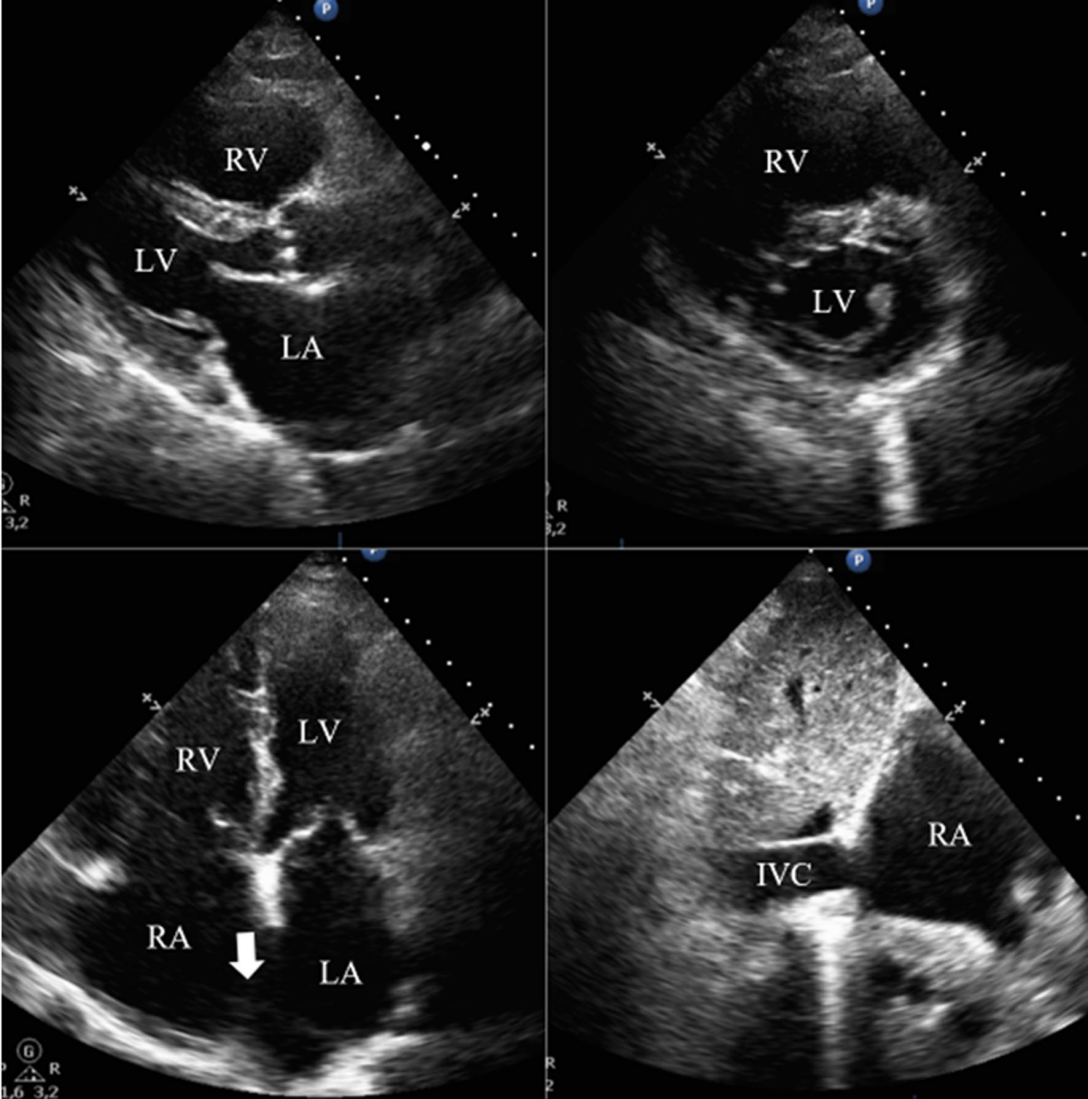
TTE imaging showing severe dilation of right cardiac chambers and RV volume overload secondary to a secundum ASD. During hospitalization, a TTE was performed demonstrating an ostium secundum ASD (white arrow) with significant left-to-right shunt (Qp:Qs = 2.6); moderate tricuspid regurgitation, estimating a PASP of 50 mmHg; severe dilation of right heart chambers and LA; dilation of IVC (27 mm) with respiratory variability < 50%; interventricular septal flattening suggesting RV volume overload; preserved global LV systolic function (+/− 63%); preserved RV systolic function; and indeterminate diastolic dysfunction. *ASD* atrial septal defect, *IVC* inferior vena cava, *LA* left atrium, *LV* left ventricle/cular, *PASP* pulmonary artery systolic pressure, *Qp:Qs* pulmonary-systemic flow ratio, *RA* right atrium, *RV* right ventricle/cular, *TTE* transthoracic echocardiography
